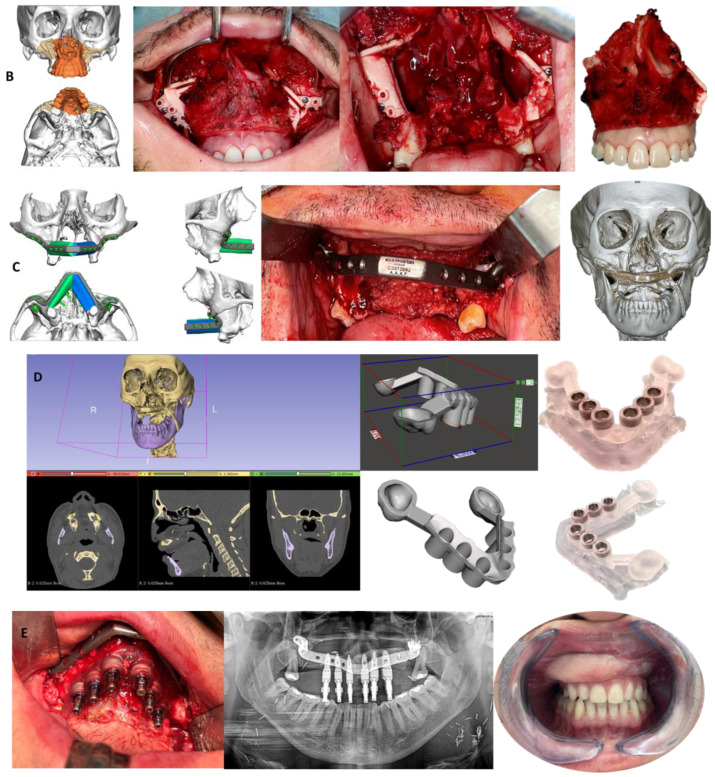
(A) Solid mass in the nasal cavity, located in the right paracentral region, diagnosed as a nasal chondrosarcoma. (B) 3D planning, virtual design of resection, in-house manufacturing of patient-specific devices and tumor resection. (C) Fibular flap harvest using CAD-CAM cutting guides, intraoperative adaptation and fixation of the fibular segments with customized plate and 3D CT postoperative. (D) Patient-specific surgical guide fabricated with SLA technology using Biomed Clear V1 material. Surgical guide designed for implant placement through static navigation. (E) Placement of six dental implants using static navigation, postoperative control by orthopantomography, and prosthetic rehabilitation within 72 h.
